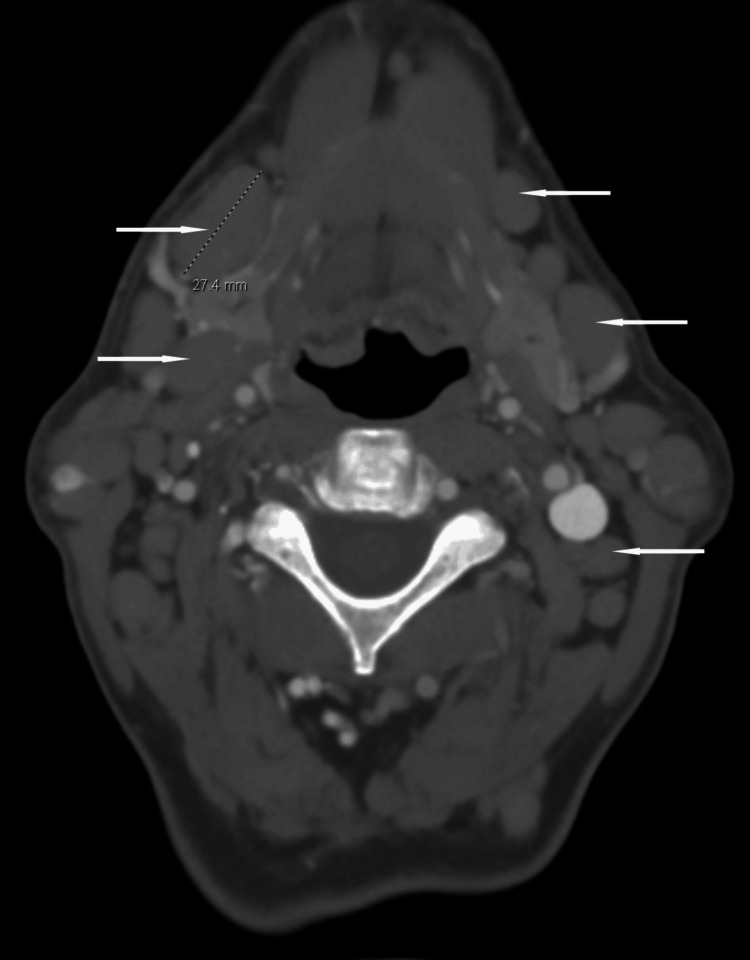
Contrast-enhanced computed tomography scan (axial view) of the head and neck showing enlarged cervical lymph nodes (arrows).
